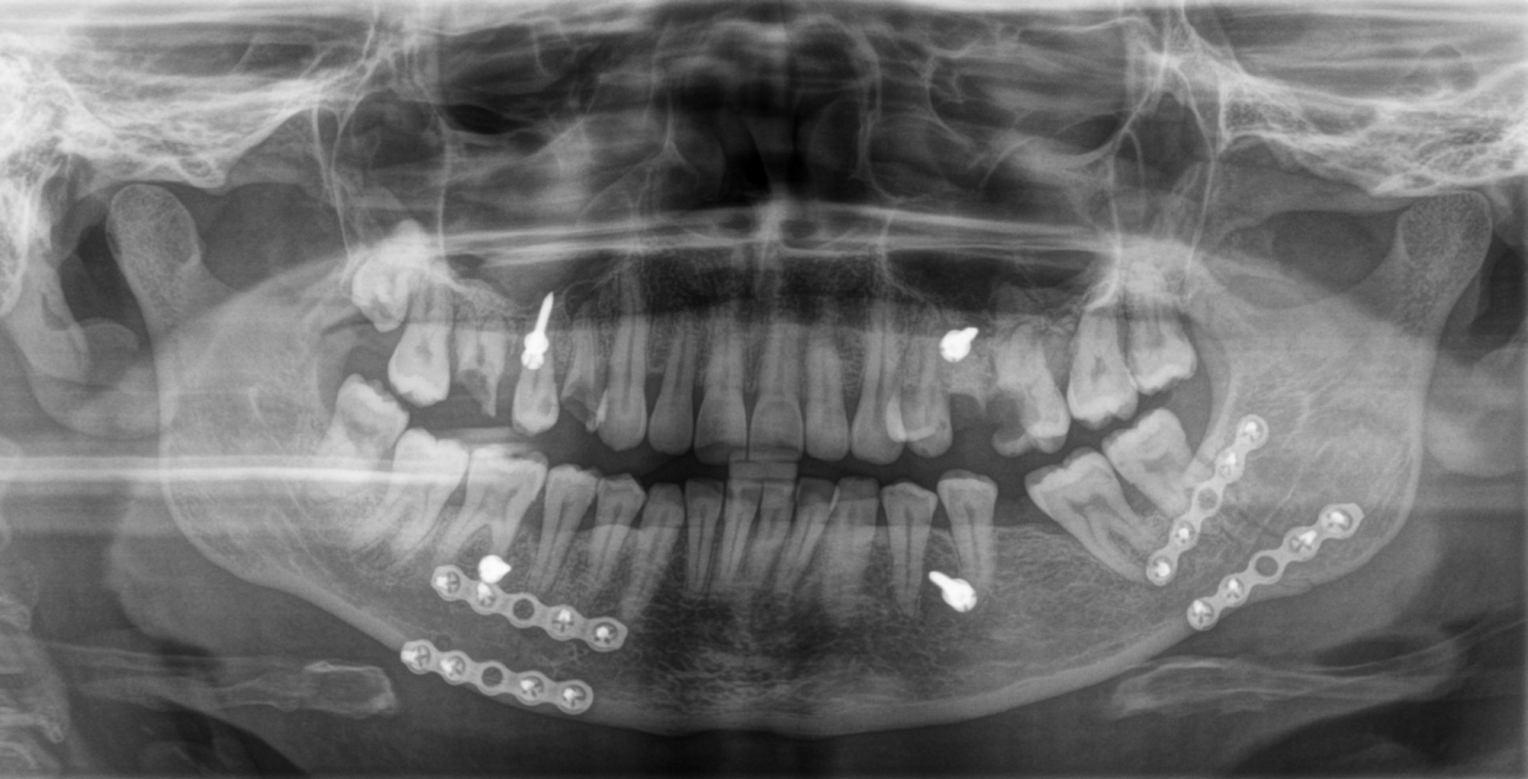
Postoperative orthopantomogram after repositioning of a left mandibular angle fracture and 2-plate fixation
